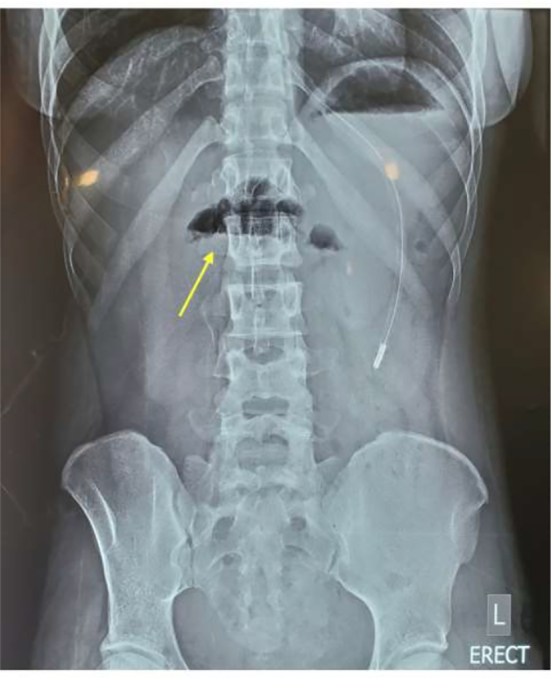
Plain erect abdominal X-ray showing air fluid level indicative of the double-bubble sign (yellow arrow). (For interpretation of the references to color in this figure legend, the reader is referred to the web version of this article.)
